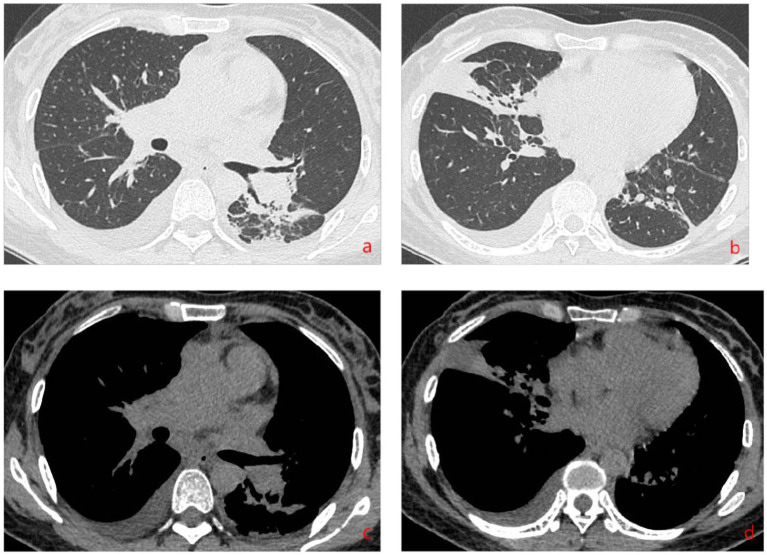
Chest CT on admission (November 12, 2024). (a–d) Show signs of *Aspergillus* infection in both lungs, small amount of pericardial effusion, multiple enlarged lymph nodes in both hilar regions and mediastinum, and small amounts of pleural effusion bilaterally.
